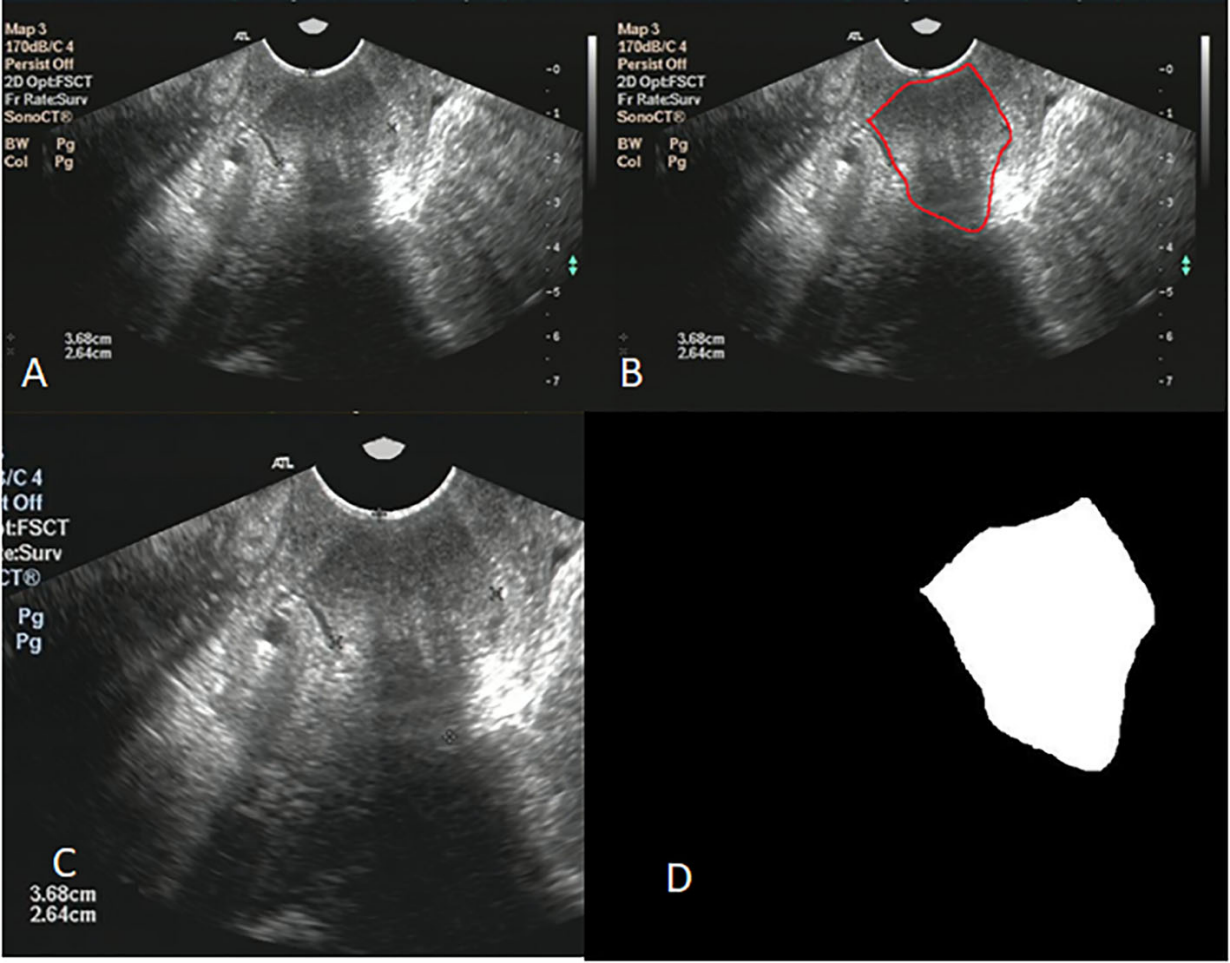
(A) shows the original ultrasound image; (B) shows ovarian tumor segmented by radiologist; (C) shows the image after clipping; (D) shows the mask of ovarian.
